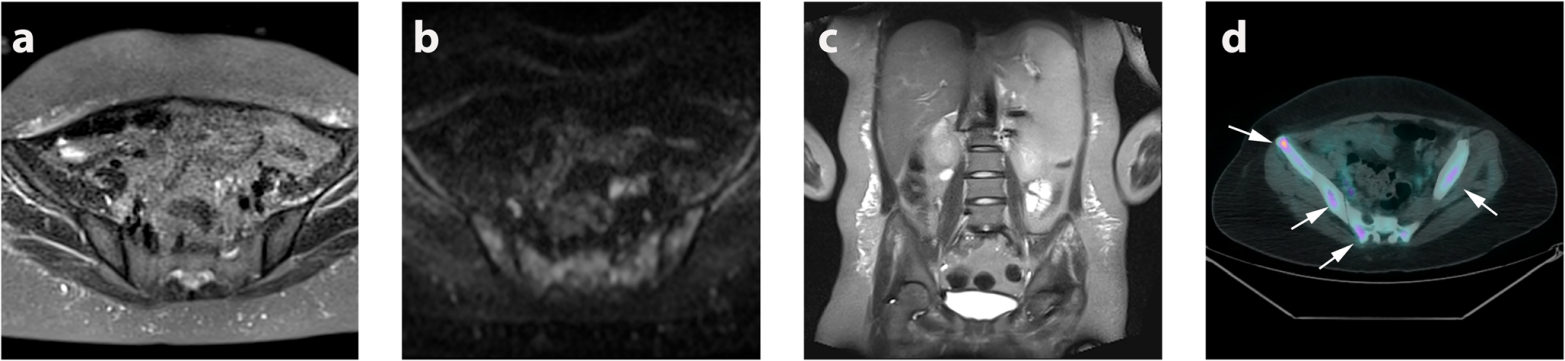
Example of WB-MRI technical error. False negative WB-MRI technical error resulting in under-staging of a 15-year-old female patient with multifocal bone marrow involvement; a axial STIR-HASTE, b DWI b_500_ and c coronal STIR-HASTE MRI show no discernible bone marrow abnormality. d
